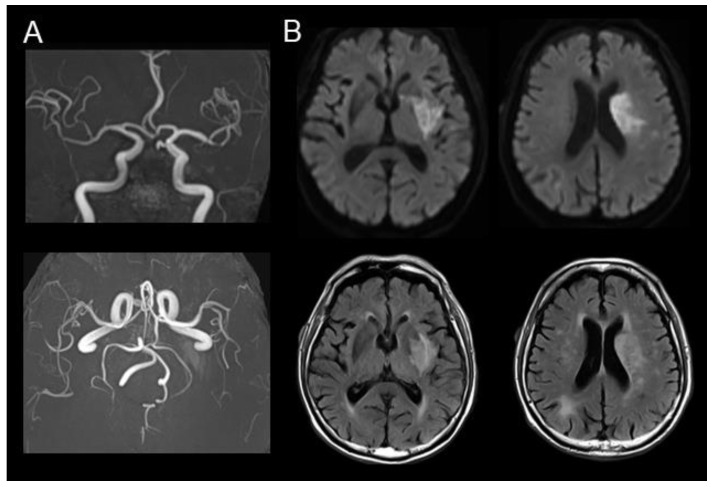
Postoperative imaging examination two days after reperfusion therapy. (A) Magnetic resonance angiography demonstrated successful recanalization of the left M1. (B) Both diffusion-weighted and fluid-attenuated inversion recovery images showed increased signals in the same area as those detected in initial MRI. Large parts of the frontotemporal gyri, including the primary sensorimotor and speech cortices, were spared on structural MRI.
